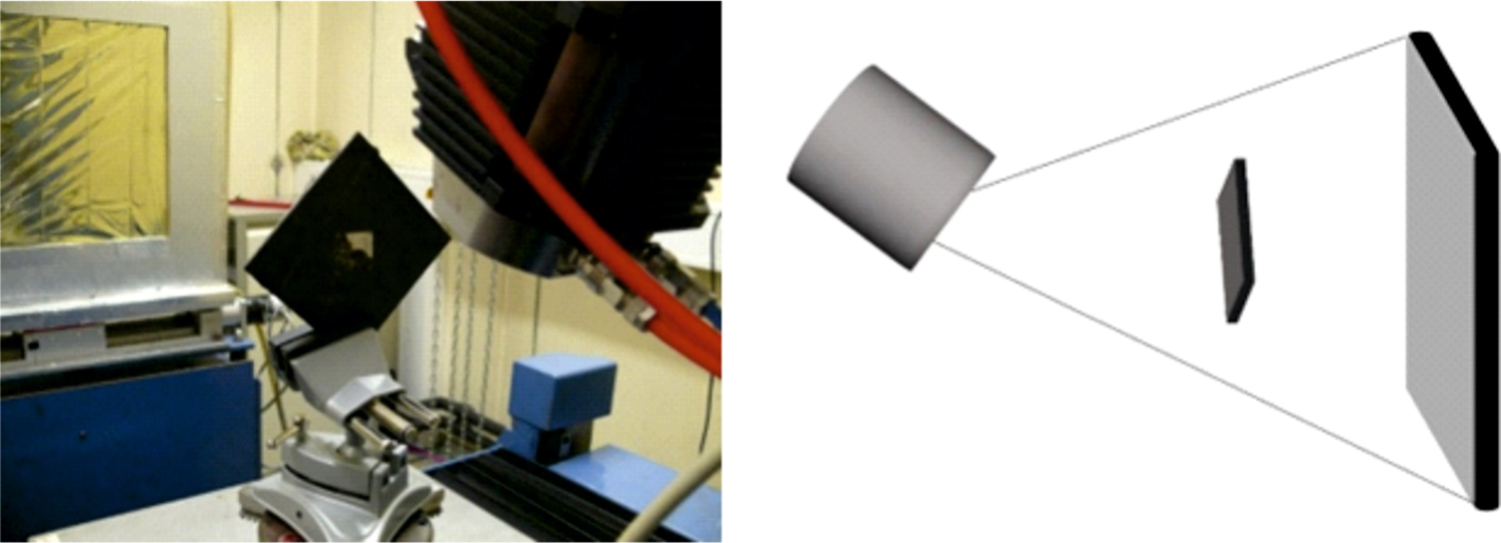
Photograph of the used CT system with the sample
